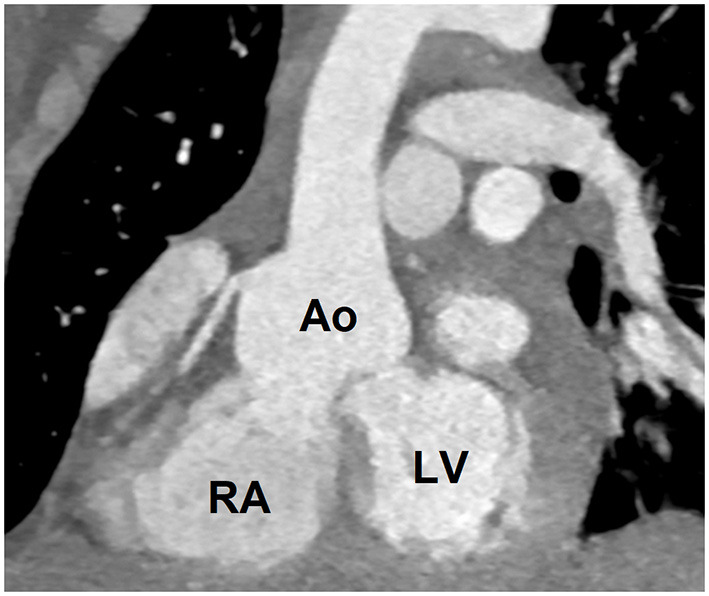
Computed tomography angiography image showing ruptured aneurysm of the right sinus of Valsalva (SVA); contrast can be seen passing from aorta to the right atrium; Ao, aorta; RA, right atrium; LV, left ventricle.
